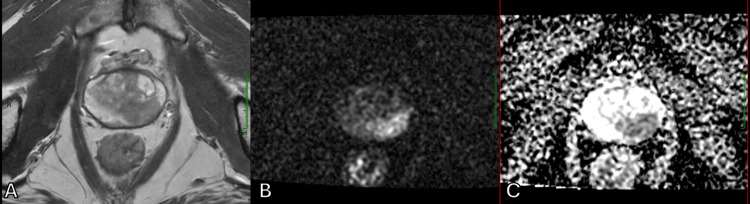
T2WI and DWI sequences, along with the ADC map, demonstrate a lentiform nodule in the left peripheral zone showing restricted diffusion and measuring 1.9/1.0 cm in the axial plane (A) Axial T2WI. (B) Axial DWI. (C) Axial ADC map. ADC, apparent diffusion coefficient; DWI, diffusion-weighted imaging; T2WI, T2-weighted imaging
